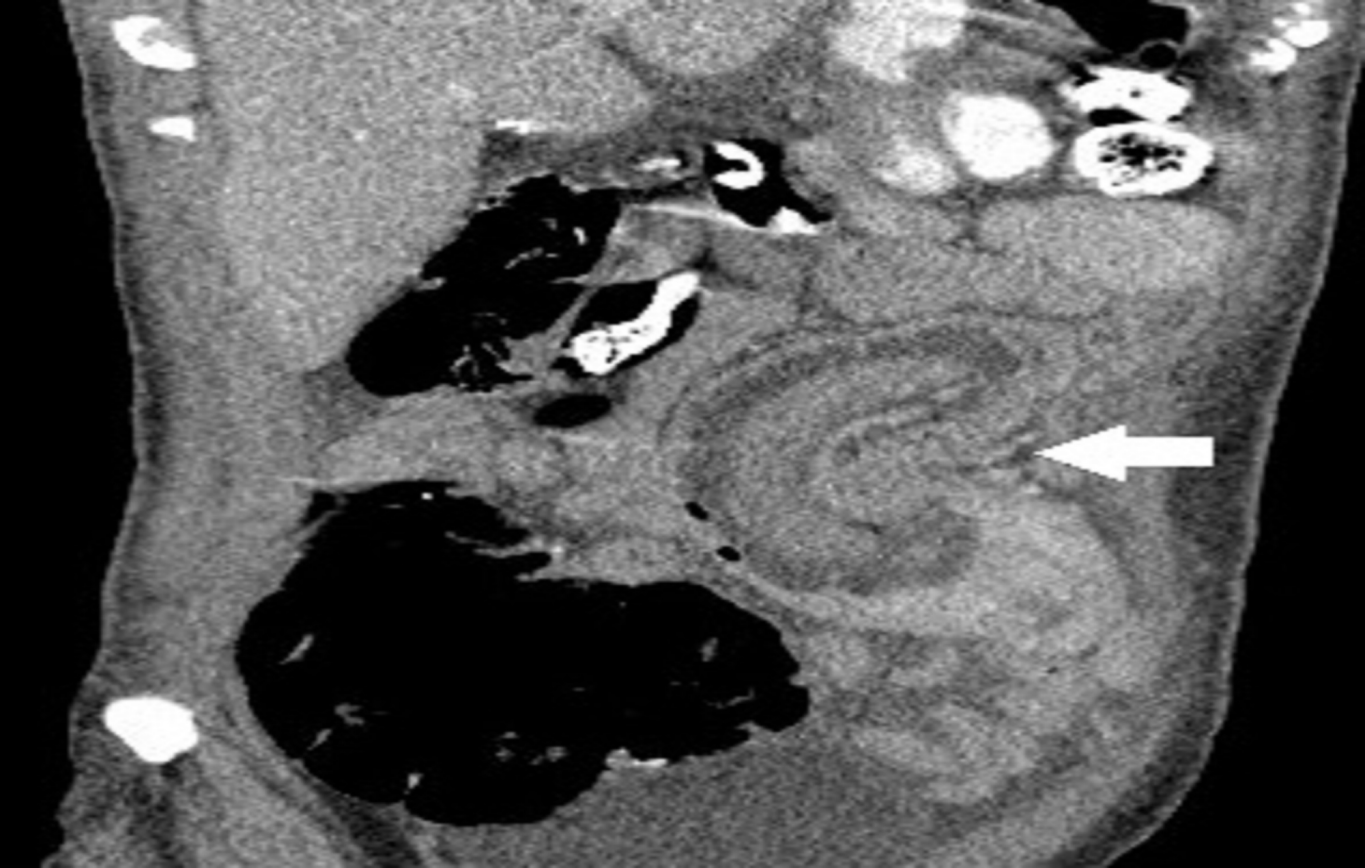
Abdominal computed tomography (CT) scan, coronal view, showing dilatation of the biliopancreatic limb of the REYGB with SB intussusception (arrow) through the jejunojejunostomy REYGB: Roux-en-Y gastric bypass surgery; SB: small bowel.
